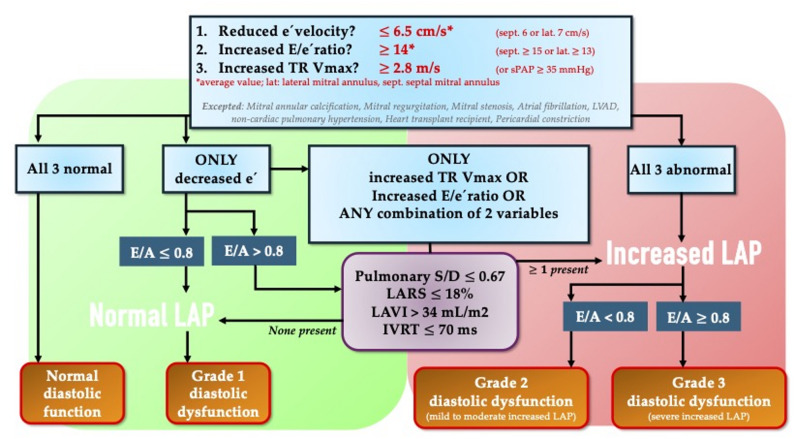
2025 ASE algorithm for the echocardiographic diagnosis and grading of left ventricular diastolic dysfunction and estimation of LAP. Diagnostic approach is based on 3 key parameters: e´ mitral annular velocity, E/e´ ratio, and TR Vmax. Additional parameters, including the E/A ratio, pulmonary vein systolic/diastolic (S/D) ratio, LAVI, LARS, and IVRT, are used to refine classification in intermediate cases (reproduced from Nagueh et al., under the terms of CC BY 4.0) (Nagueh et al. 2025). ASE: American Society of Echocardiography; IVRT: Isovolumetric relaxation time; LAP: Left atrial pressure; LARS: Left atrial reservoir strain; TR Vmax: Peak tricuspid regurgitation velocity
